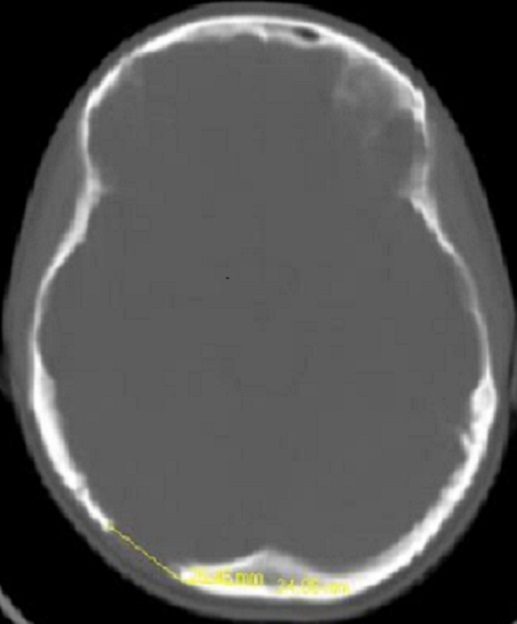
Cranial bone CT image of 3x3 cm bone defect on right occipital bone
